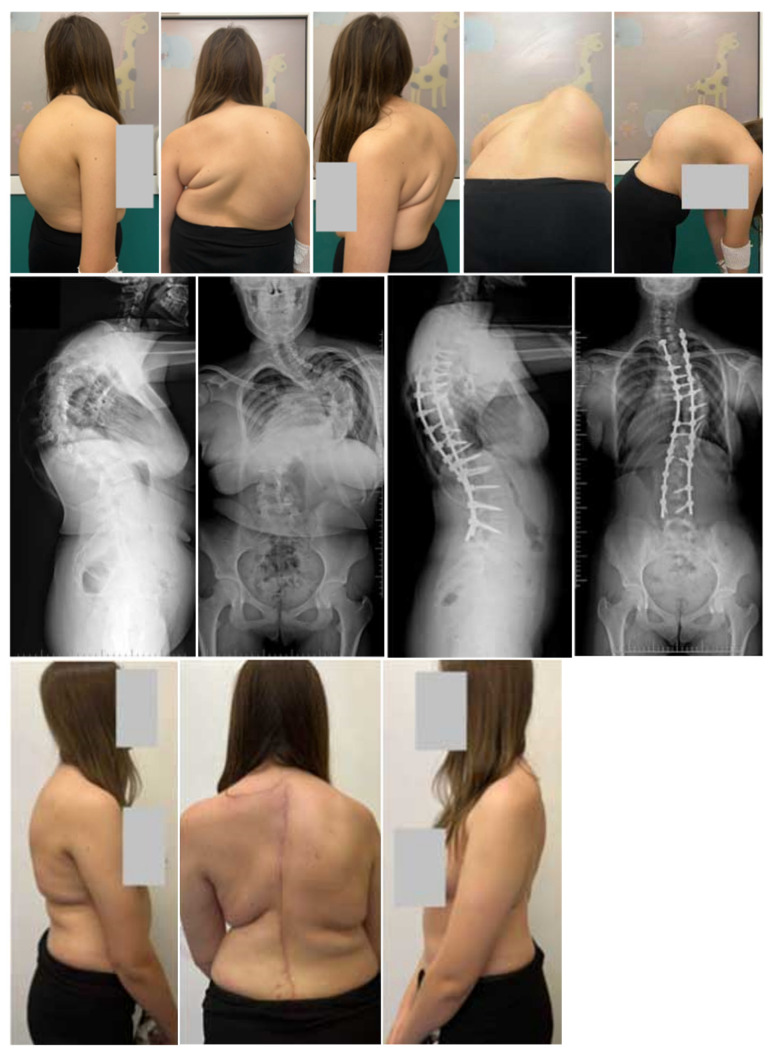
A 14-year-old female patient treated with preoperative HGT followed by posterior column osteotomies and PSF. Clinical photographs and radiographs performed pre- and postoperatively at final follow-up.
